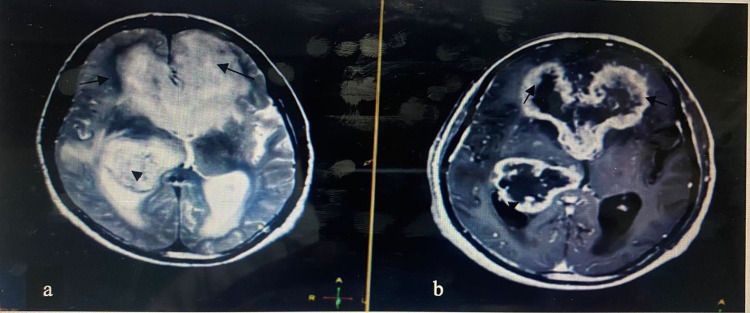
MRI brain of a 70-year-old male showing high-grade glioma (GBM) seen involving the bilateral frontal horns (arrows in a and b) and body and occipital horns (arrowheads in a and b) appearing high on T2WI and showing significant post-contrast enhancement on T2WI in b. MRI: magnetic resonance imaging; GBM: glioblastoma; T2WI: T2-weighted images
